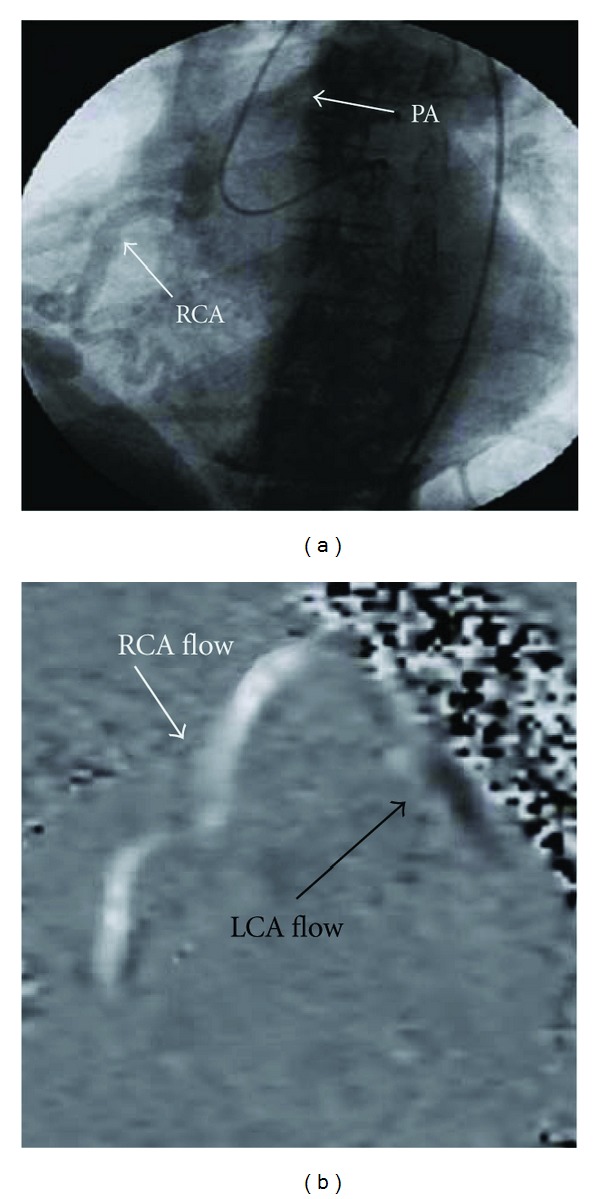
(a) Coronary angiogram. The catheter is in the aorta and the left main is engaged. Collateral vessels from the LCA deliver contrast media into the RCA, which subsequently drains into the main PA. (b) MRI velocity flow map. Coded black is flow down the LCA away from the aorta. Coded white is flow up the RCA towards the main PA. PA: pulmonary artery; RCA: right coronary artery; LCA: left coronary artery.
